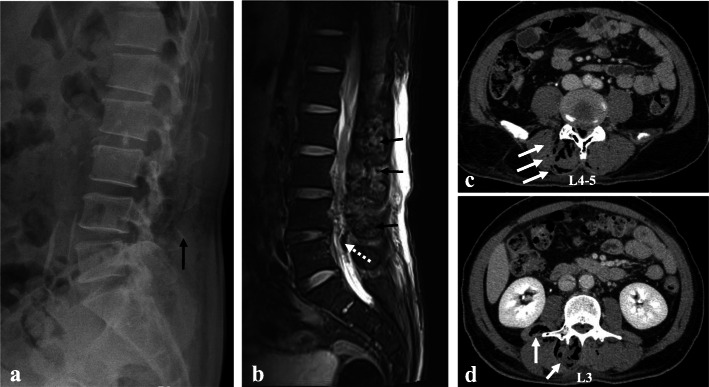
Lateral radiographs (a) showing a small gas-forming lesion around the lumbar paravertebral area (black arrow). T2-weighted sagittal magnetic resonance imaging (b) showing multifocal fluid pockets containing air bubbles in the paraspinal area (black arrows), whole lumbar area, and epidural space (white dotted arrow). Computed tomography (c and d) scan revealing subcutaneous emphysema in the right paravertebral muscles including the multifidus and quadratus lumborum, mainly in the right side (white arrows), as well as the lumbar spinal canal at L2-S1
